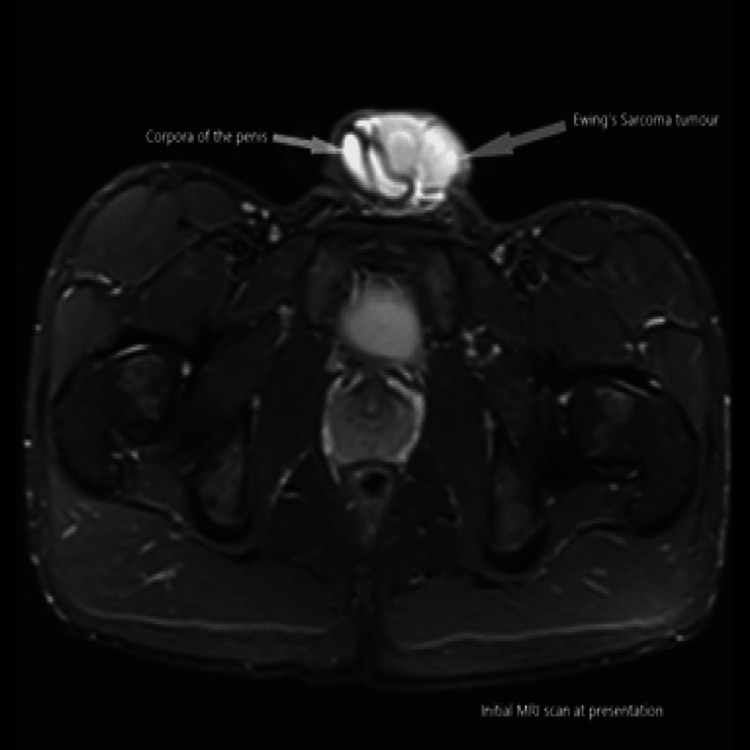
Initial MRI scan at presentation (axial plane)
